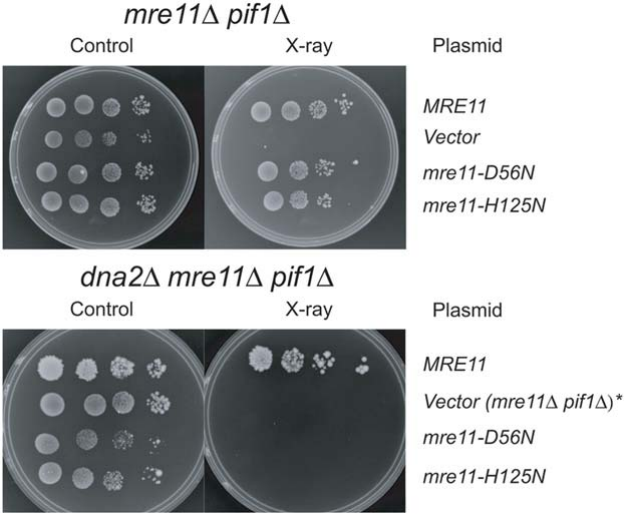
dna2Δ pif1Δ mre11Δ* (pRS414*mre11-D56N*) and *dna2Δ pif1Δ mre11Δ* (pRS414*mre11-H125N*) strains exhibit similar sensitivity to X-rays as *mre11Δ pif1Δ*. These strains were created by dissecting tetrads from the following crosses: *MATa dna2Δ pif1Δ trp1Δ bar1Δ*/ *MATα mre11Δ trp1Δ* (pRS414*MRE11*), *MATa dna2Δ pif1Δ trp1Δ bar1Δ/ MATα mre11Δ trp1Δ* (pRS414*mre11-D56N*) and *MATa dna2Δ pif1Δ trp1Δ bar1Δ*/ *MATα mre11Δ trp1Δ* (pRS414*mre11-H125N*) diploids. Trp^+^ spores were replica plated, and irradiated. Control strains *mre11Δ pif1Δ* (pRS414*MRE11*) and *dna2Δ pif1Δ mre11Δ* (pRS414*MRE11*) were identified from the respective cross and were X-ray resistant. *dna2Δ pif1Δ mre11Δ* (pRS414*mre11D56N*) or *dna2Δ pif1Δ mre11Δ* (pRS414*mre11H125N*), from the two respective crosses were radiation sensitive. To compare the radiation sensitivity of the *dna2Δ pif1Δ mre11Δ* (pRS414*mre11-D56N*) and the *dna2Δ pif1Δ mre11Δ* (pRS414*mre11-H125N*) (bottom panel) to *mre11Δ pif1Δ* (pRS414*mre11-D56N*) and to *mre11Δ pif1Δ* (pRS414*mre11-H125N*) strains (top panel), serially diluted cells were irradiated with 26 krads on a plate and allowed to grow for three days. Note that a *dna2Δ pif1Δ mre11Δ* strain carrying only the pRS vector is inviable, so an *mre11Δ pif1Δ* strain carrying the pRS vector alone is shown as the control, as indicated by the * in the bottom panel.
